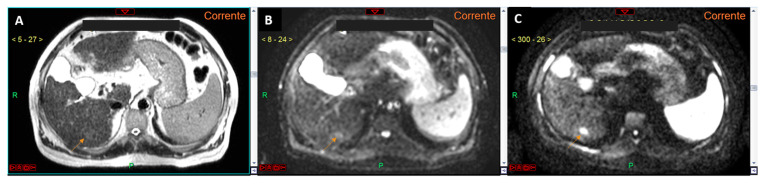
T2-weighted image (A), diffusion-weighted image with a b-value 0 (B), and diffusion-weighted image with a b-value 800 (C). The lesion located in the liver segment VI is clearly visible (arrows in (A–C)). It is evident that the conspicuity (hyperintensity) of the lesion is greater in the b-value 800 image (C). Moreover, the hyperintensity of the lesion in the b-value 0 image (B) is more pronounced compared to the T2-weighted image (A), confirming our hypothesis that the use of the b-value 0 could replace the T2-weighted sequence in aMRI protocols.
